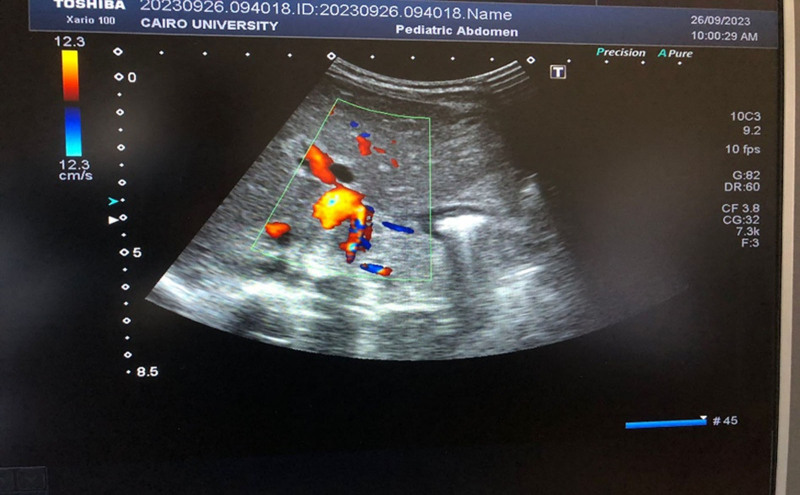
Color Doppler ultrasound scan showing portal cavernoma.
